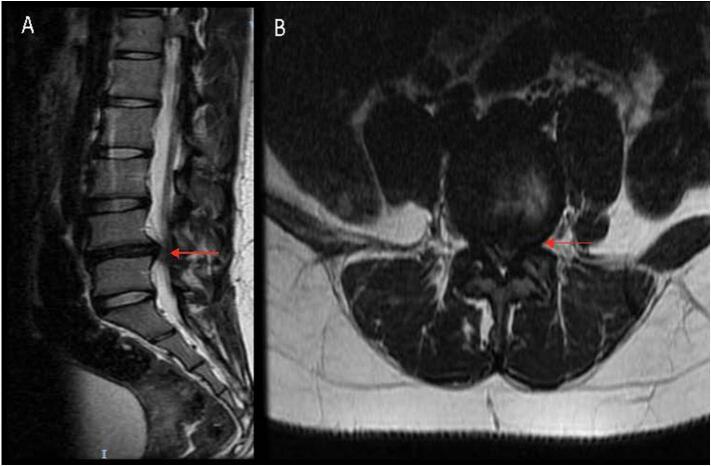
Patient 5: Preoperative MRI. (A) Sagittal T2-weighted MRI showing disc protrusion at the L4–5 level. (B) Axial T2-weighted image showing stenosis at the same level.
